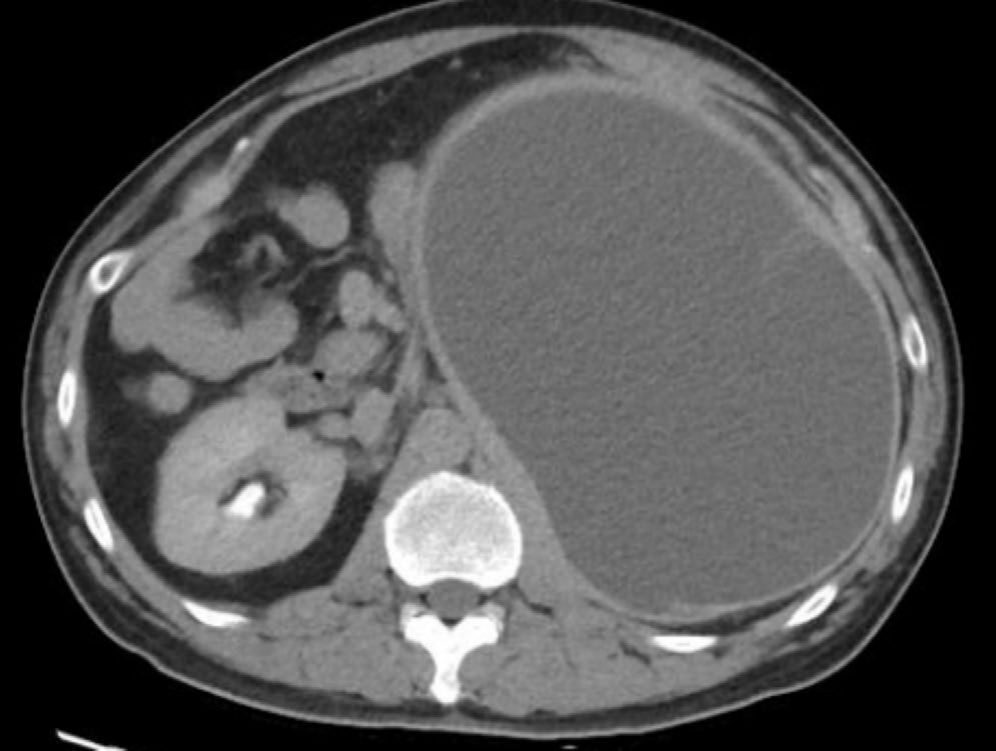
CT examination of the abdomen shows a large peripancreatic pseudocyst extending into the left perinephric region.
